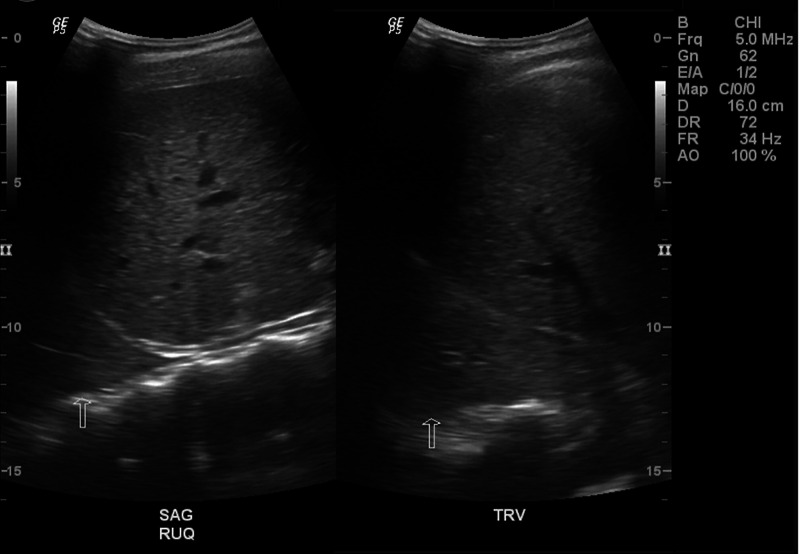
Ultrasound chest There are small bilateral pleural effusions. The fluid appears to be complex
